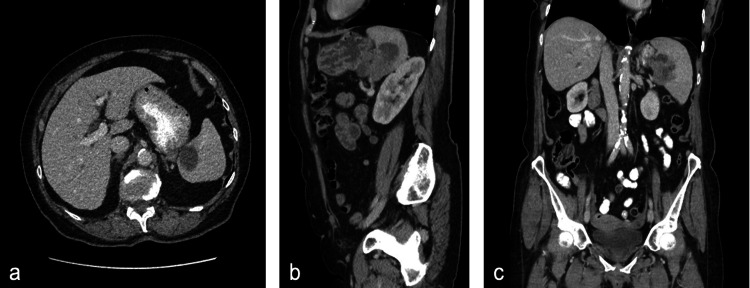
Axial (a), sagittal (b), and coronal (c) planes of CT showing a complex mass with a cystic component arising from the splenic hilum.
